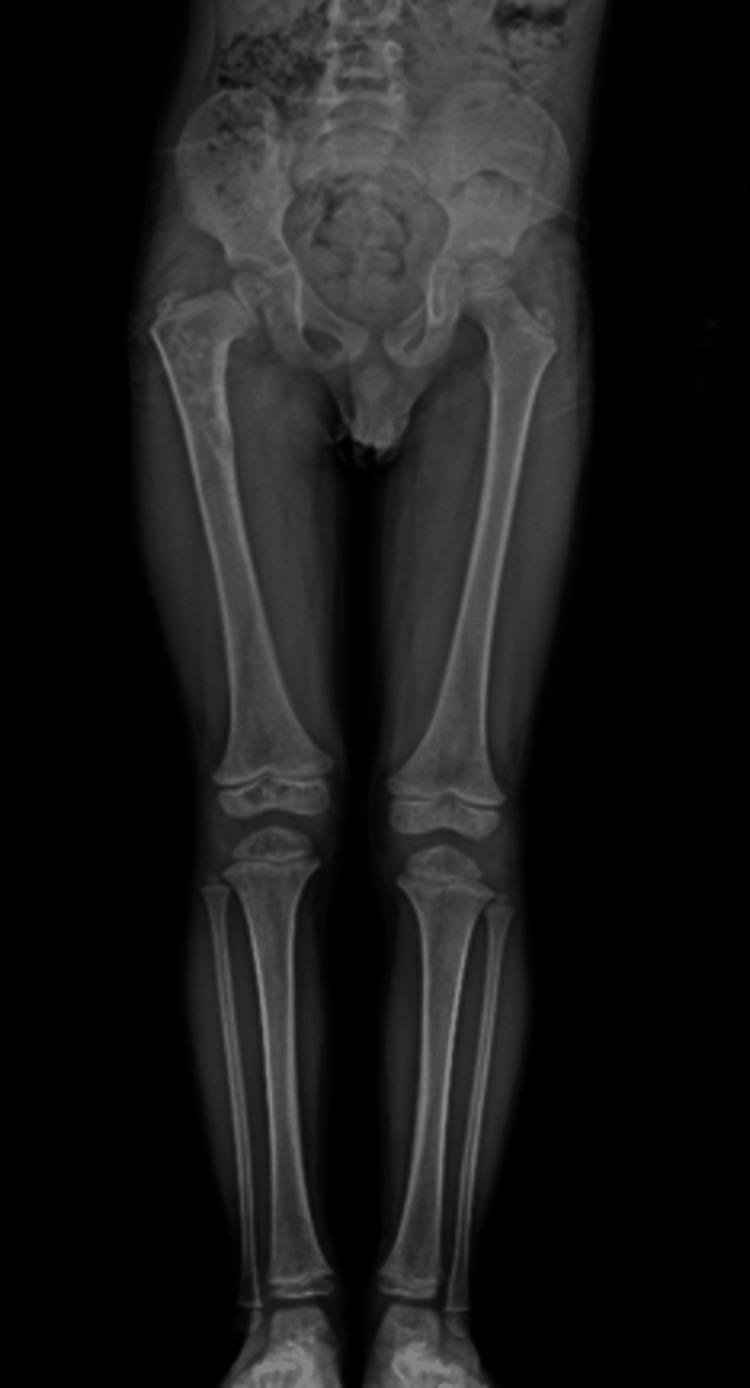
There is no evidence of an aneurysmal bone cyst on the third-year follow-up radiograph, but he is followed up because of coxa vara
